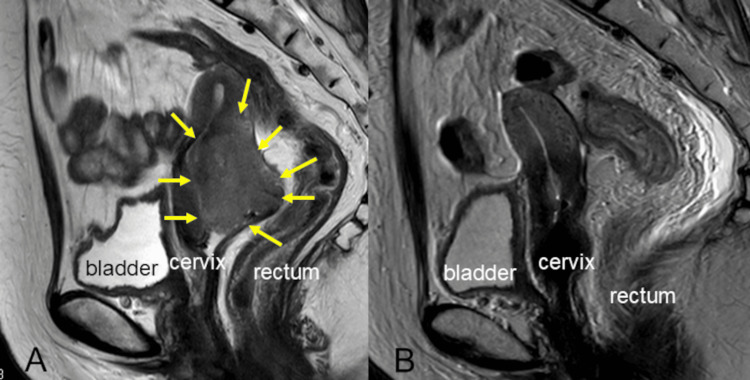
MRI images before and after primary treatment A: Before primary CCRT; B: After primary CCRT; Yellow arrow: cervical cancer CCRT: concurrent chemoradiotherapy
